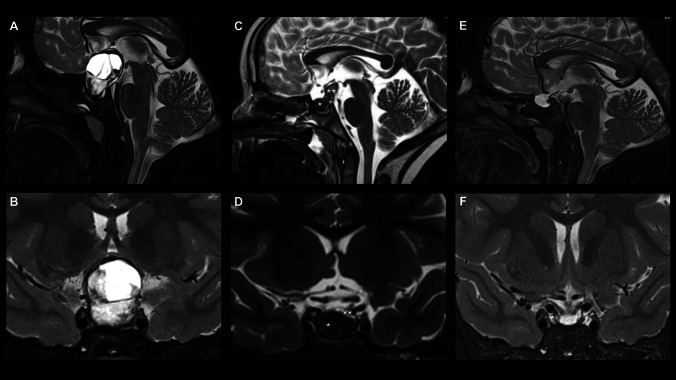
Radiological course. A Preoperative sagittal and B coronal T2-weighted MRI of the cystic sellar and suprasellar infantile mature teratoma. C Immediate postoperative sagittal and D coronal T2-weighted MRI demonstrating skull base defect reconstruction material within the sphenoid cavity and the sella turcica [*], along with a decompressed and anatomically located optic chiasm and remnant solid tumor supero-posteriorly []. E 1-year postoperative sagittal and F coronal T2-weighted MRI demonstrating resorption of the intrasellar reconstruction material along with volumetric regression and descent of the solid tumor remnant along the pituitary stalk into the dorsal aspect of the sella turcica. The optic nerves and chiasm descent into the sellar region and remains decompressed and anatomically preserved
